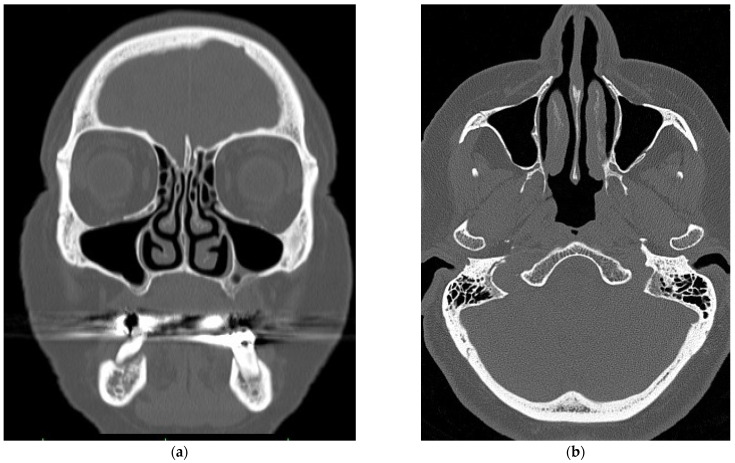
CT images of the nasal cavity—(a) frontal section (b) transverse section.
